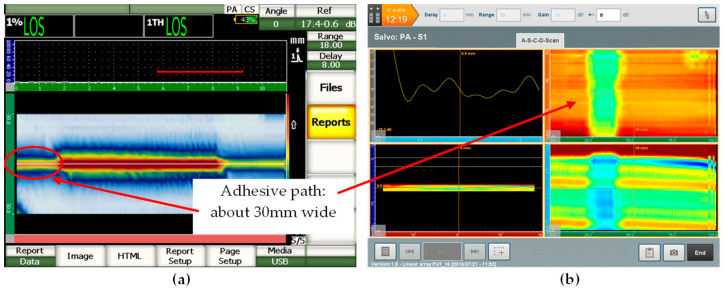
Results of ultrasonic test: (a) c-scan, (b) surface scan.
